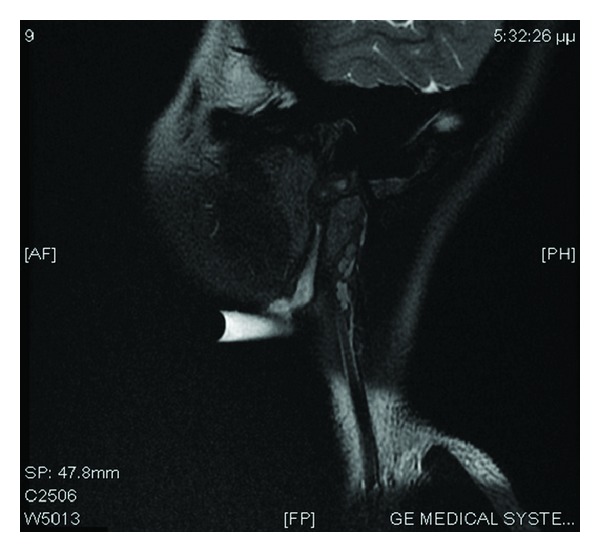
Lateral view T1-contrast enhanced MRI demonstrating the fistula and its extension to the acoustic meatus.
